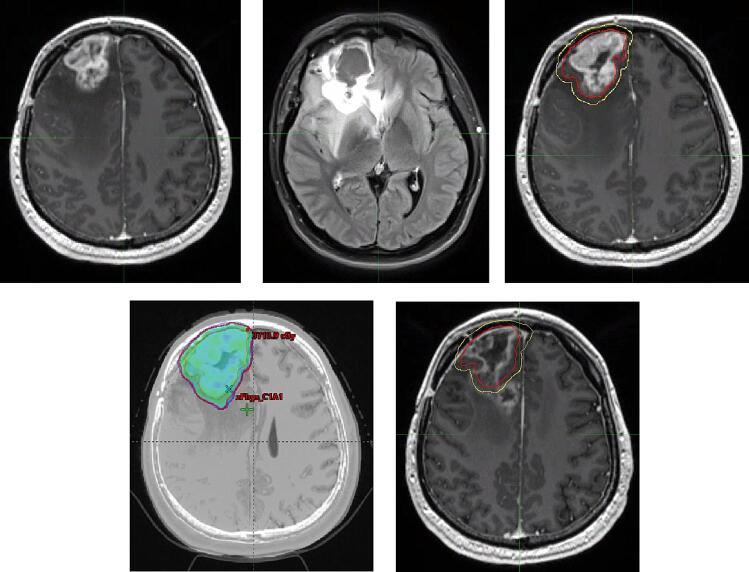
Case example of a marginal recurrence after re-RT. This 29-year-old male was re-irradiated for a right frontal recurrence of a glioblastoma about 10 months after initial diagnosis and treatment. At the time of re-treatment, the patient had already received Bevacizumab. (A) and (B) show 3D AX T1 GAD and 3D AX T2 FLAIR images at recurrence, respectively. The patient was then re-irradiated with 35 Gy/10fx concurrent with Bevacizumab and immune checkpoint inhibition. (C) shows the PTV and CTV contours, (D) shows the 95 % isodose line of IMRT re-irradiation plan. Ten months after re-RT, the patient relapsed again. The progressive, non-nodular enhancement on (E) 3D AX T1 Gad was abutting the re-RT PTV and considered a “marginal recurrence” in this study.
